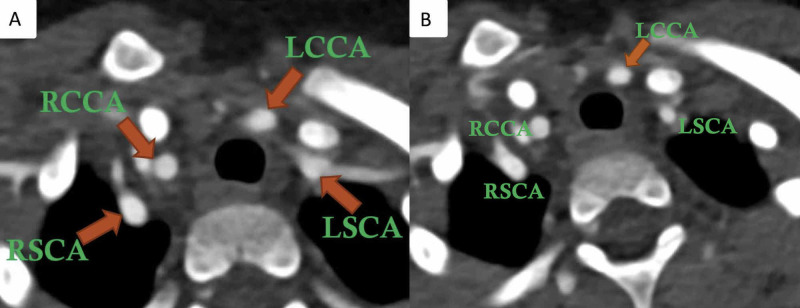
Right aortic arch branching pattern Axial CTA (A) image in a patient with a right aortic arch and mirror-image branching shows a non-symmetrical arrangement of aortic branches. Axial CTA image in the same patient as (A) shows the anterior course of the left common carotid artery. RSCA: Right subclavian artery; LSCA: Left subclavian artery; RCCA: Right common carotid artery; LCCA: Left common carotid artery
